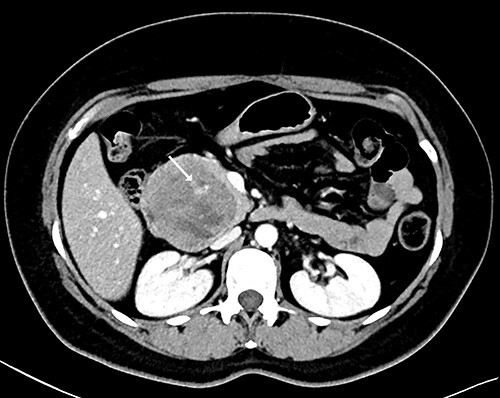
CT scan showing a well-defined heterogeneous 6.7 cm mass with solid and cystic components arising from the head of the pancreas with enhancement of contrast in the solid component, and some areas with active bleeding (white arrow). The tumour was in direct proximity to the duodenum.
